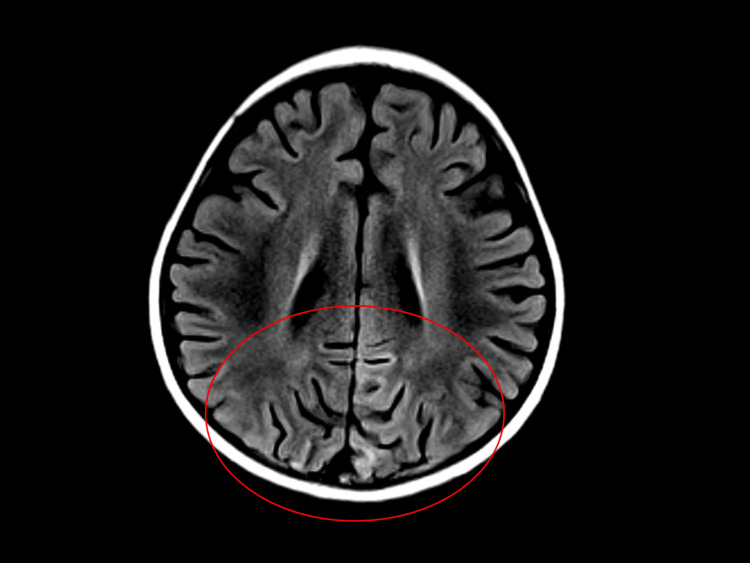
Brain FLAIR magnetic resonance imaging Hypertense signals in the cortical and subcortical white matter of bilateral occipital lobes (red circle) in a FLAIR sequence. FLAIR, fluid-attenuated inversion recovery
